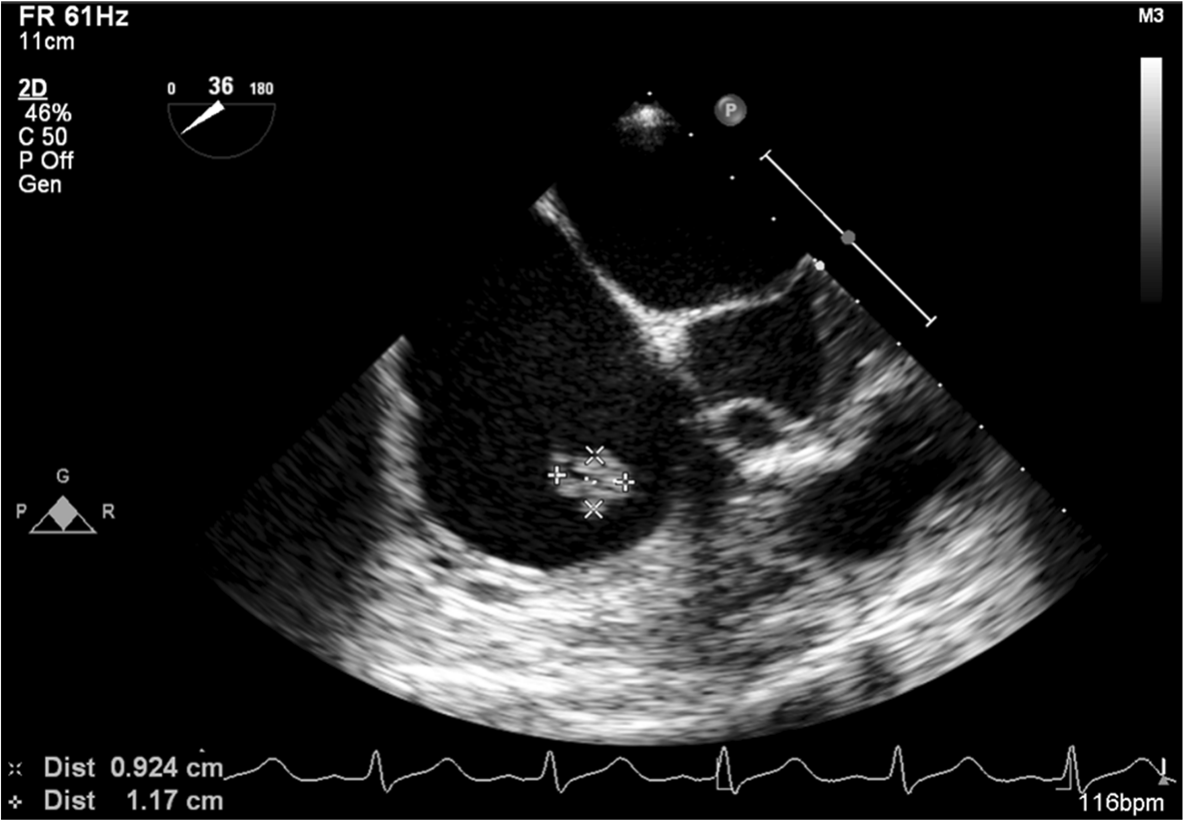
The pre-operative transesophageal echocardiography (TEE) showed 0.9 × 1.2 cm^2^ vegetation over tricuspid valve
